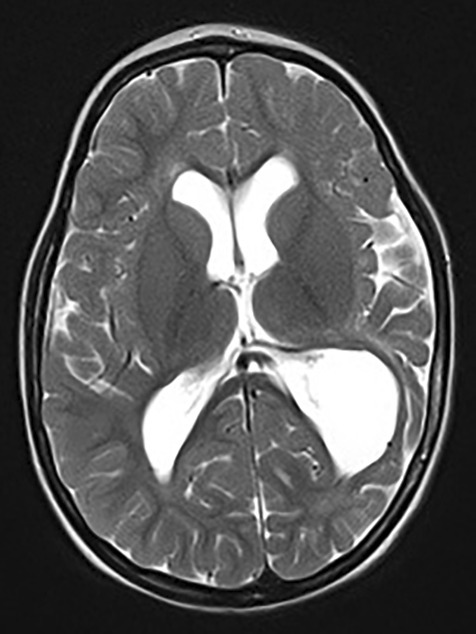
Brain MRI demonstrating atrophy of the left temporoparietal lobe 2 years after the trauma
